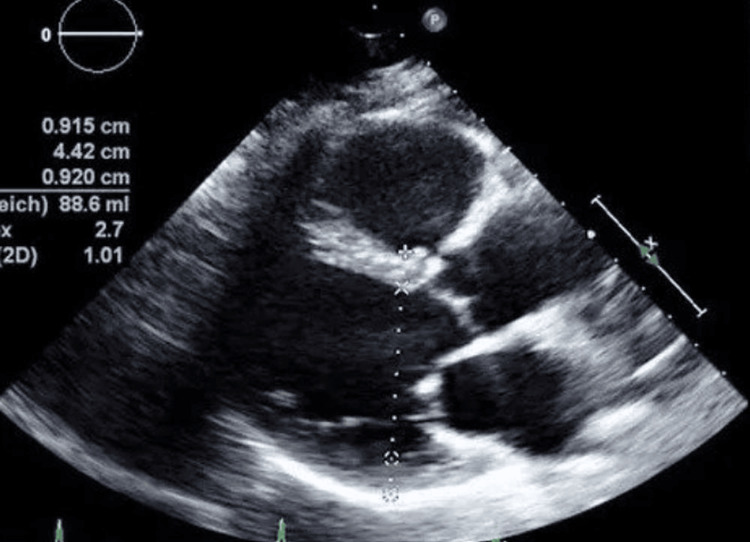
Transthoracic echocardiogram (TTE) showed normal left ventricular ejection fraction (LVEF) and no evidence of regional wall motion abnormalities (RWMA)
